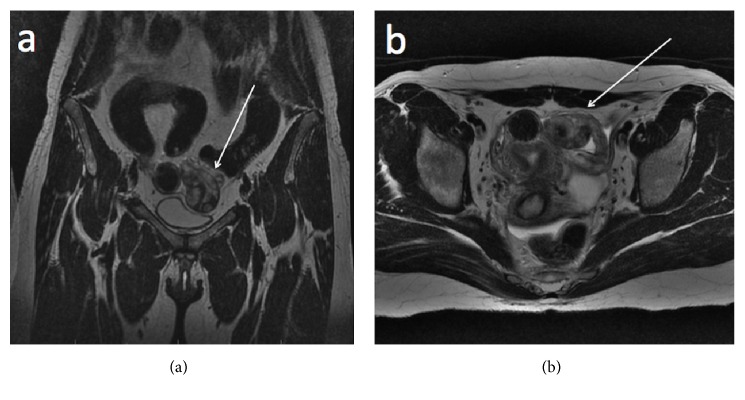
(a) T1-weighted coronal and (b) axial pelvic magnetic resonance imaging showing a cystic-solid lesion originating from the left fallopian tube (white arrow).
